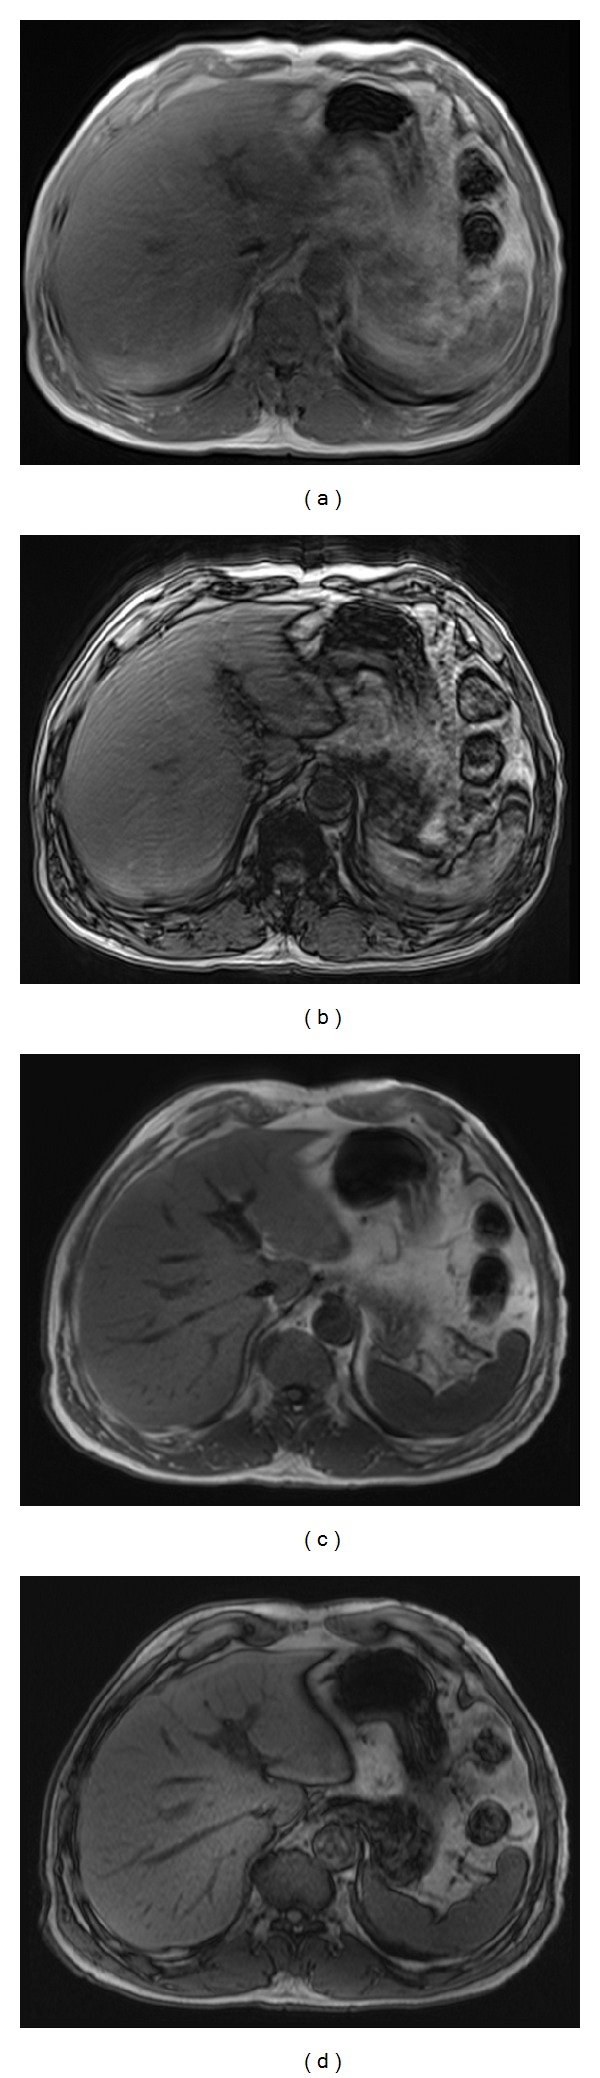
MR images obtained in a 67-year-old man. Standard T1-weighted 2D SGE in-phase (a) and out-of-phase (b) images show severe motion artifacts caused by his breathing. The motion-resistant protocol using axial T1-weighted 2D MP-RAGE in-phase (c) and T1-weighted 2D MP-RAGE out-of-phase (d) images demonstrate substantially reduced artifacts and motion-free, high-quality images in this patient.
